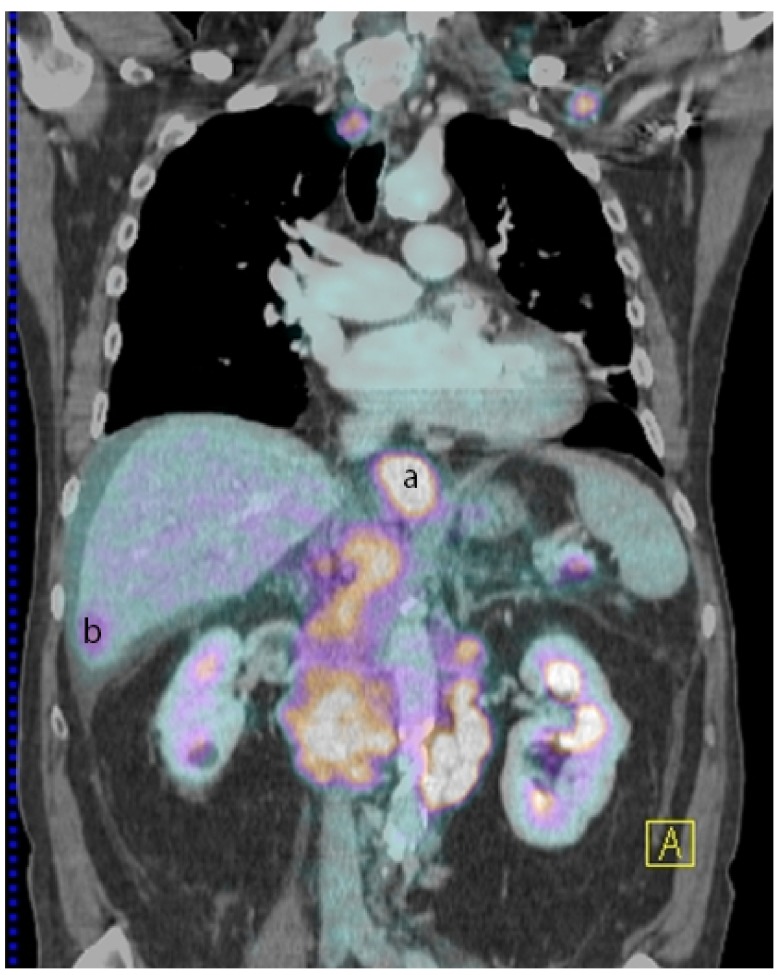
PET/CT, performed after administration of 311 MBq of Fludoxyglucose, showed increased activity in the lower oesophagus (
